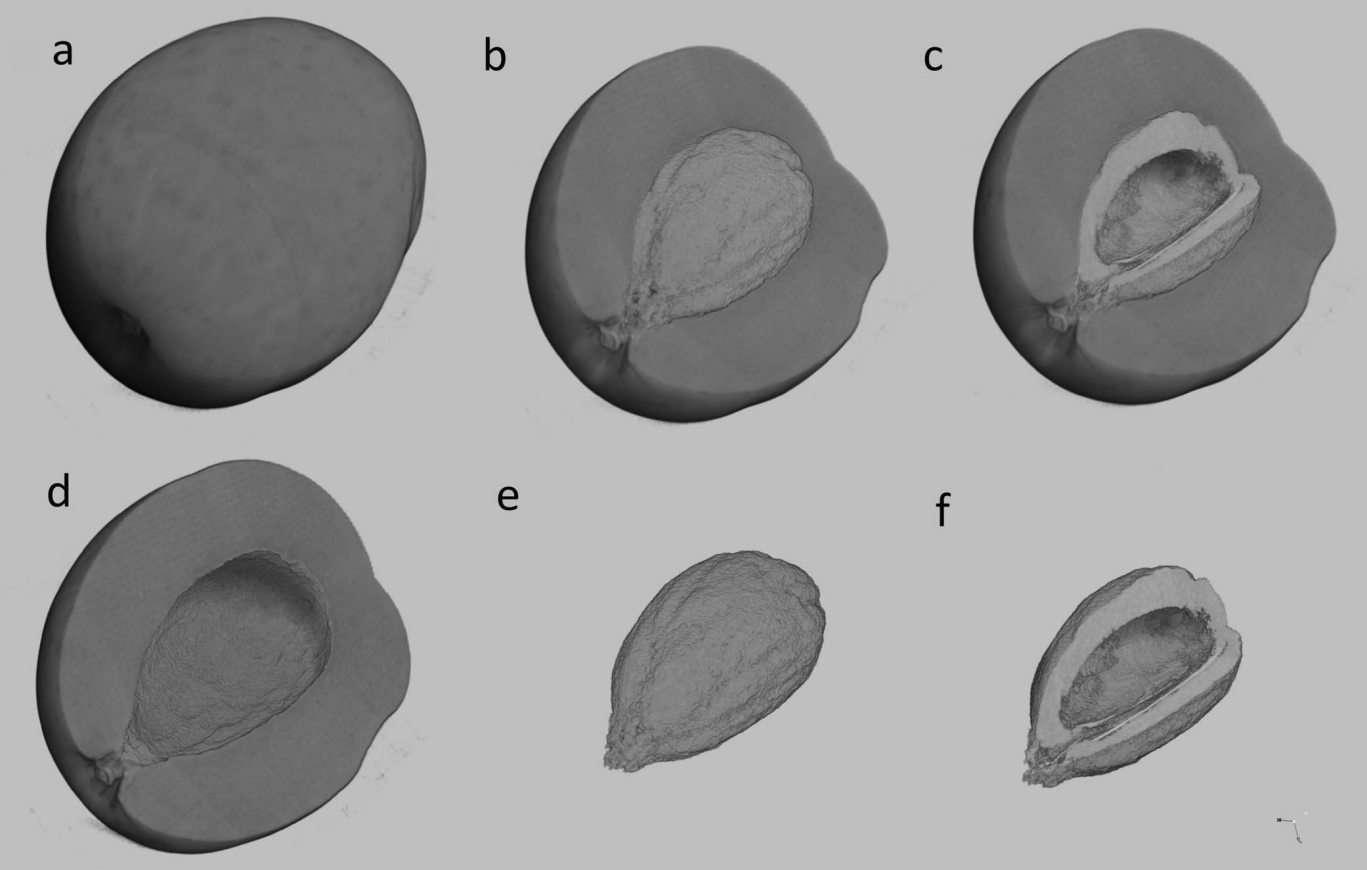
3D reconstruction of an olive and its internal parts with X‐ray micro‐CT. (a) Whole fruit; (b) sectioned mesocarp and stone; (c) sectioned mesocarp and sectioned stone with its internal cavity; (d) sectioned mesocarp without stone; (e) whole stone; (f) sectioned stone with its internal cavity.
